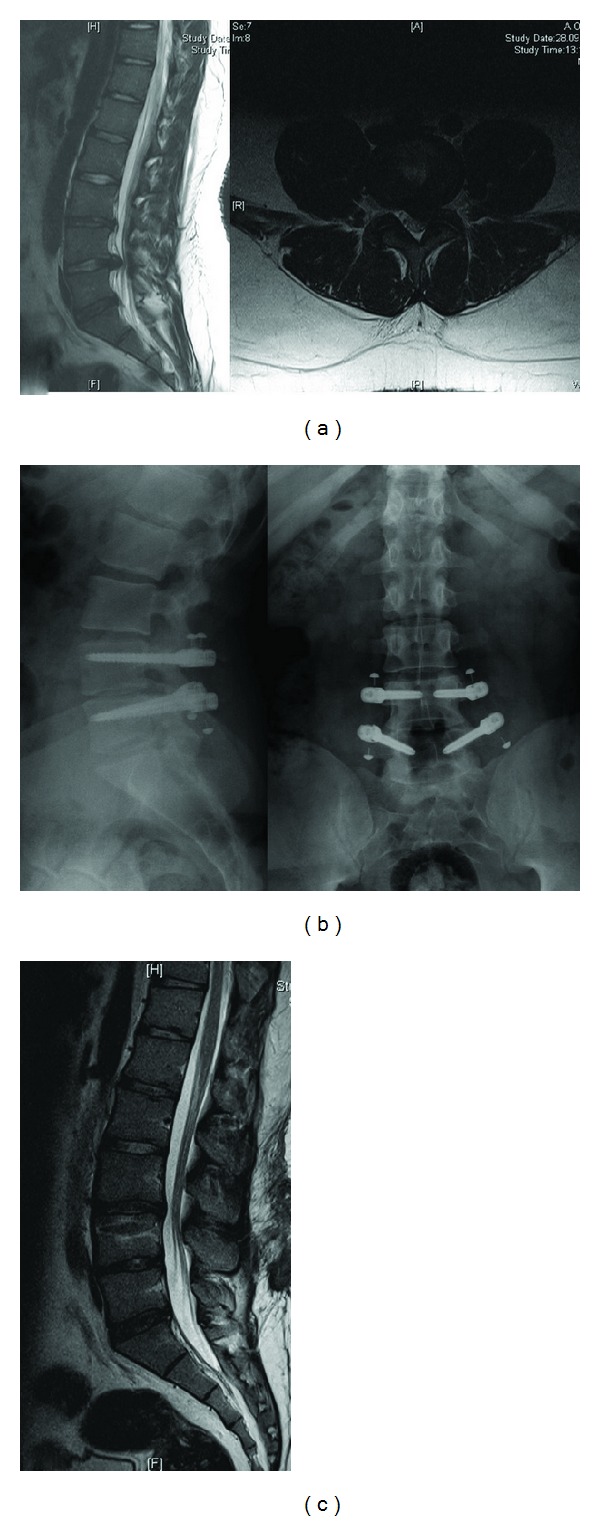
20-year-old male patient with left leg pain and back pain. (a) T2 weighted MRI scans show L4-5 disc herniation; (b) PEEK rod was applied; (c) the patient was in very good condition, and T2 weighted MRI scan showed a nearly normal appearance after one year.
